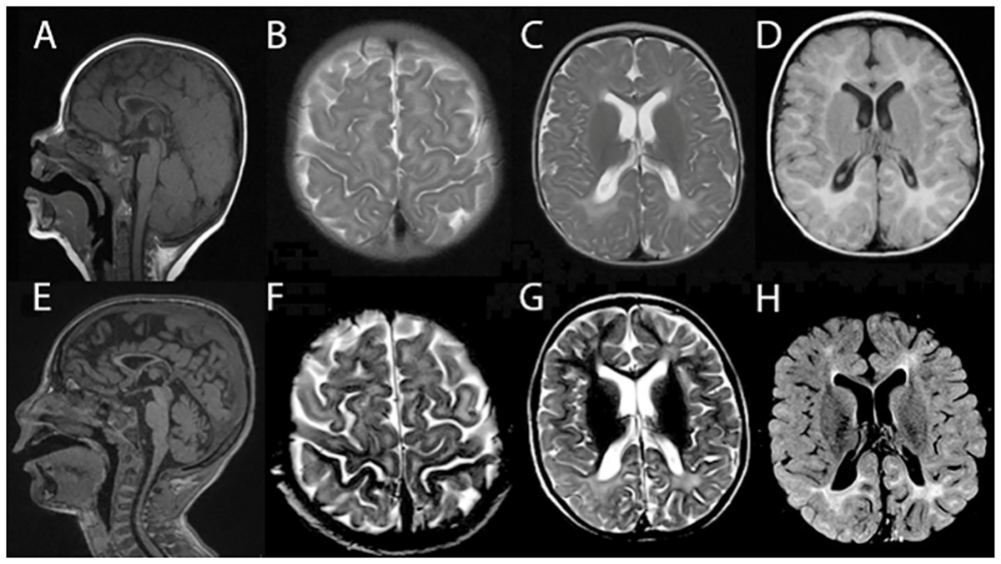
Brain MRI demonstrated white matter abnormality suggesting leukoencephalopathy in a patient homozygous for *VPS11*: c.2536T>G (p.C846G). In patient B at nine months of age, thin corpus callosum (A), T2 diffuse hyperintensity in the peri-Rolandic white matter (B) and posterior occipital white matter region (C) were seen; FLAIR diffuse hyperintensity in the white matter region was also seen (D). At five years of age, diminutive corpus callosum (E), T2 diffuse hyperintensity signal in peri-Rolandic areas (F) and supratentorial white matter, most pronounced within the bilateral parieto-occipital regions (G) were seen; FLAIR diffuse hyperintensity in the white matter region with a mild increase of myelination was seen(H).
